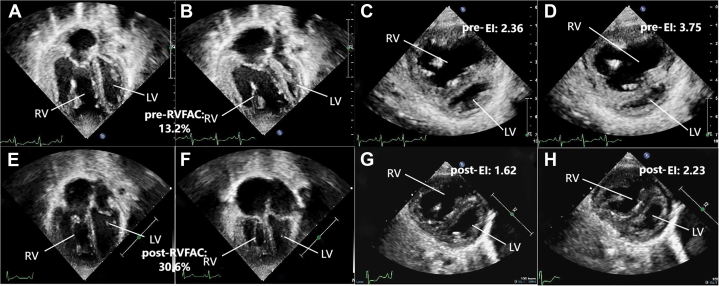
Echocardiographic Assessment of Left and Right Ventricular Function Before and After Surgery (A to D) Preoperative images and (E to H) images 6 months postoperative are shown. (A and B) Apical 4-chamber view showing ventricular size and interventricular septum status at end-diastole and end-systole preoperatively, with RVFAC measured at 13.2%. (C and D) Left ventricular short-axis view showing end-diastolic eccentricity index of the left ventricle (EID) and end-systolic eccentricity index of the left ventricle (EIS) preoperatively, with EID of 2.36 and EIS of 3.75. (E and F) Postoperative apical 4-chamber view showing RVFAC of 30.6%. (G and H) Postoperative left ventricular short-axis view showing EID of 1.62 and EIS of 2.23. EI = eccentricity index; LV = left ventricle; RV = right ventricle; RVFAC = right ventricular fractional area change.
